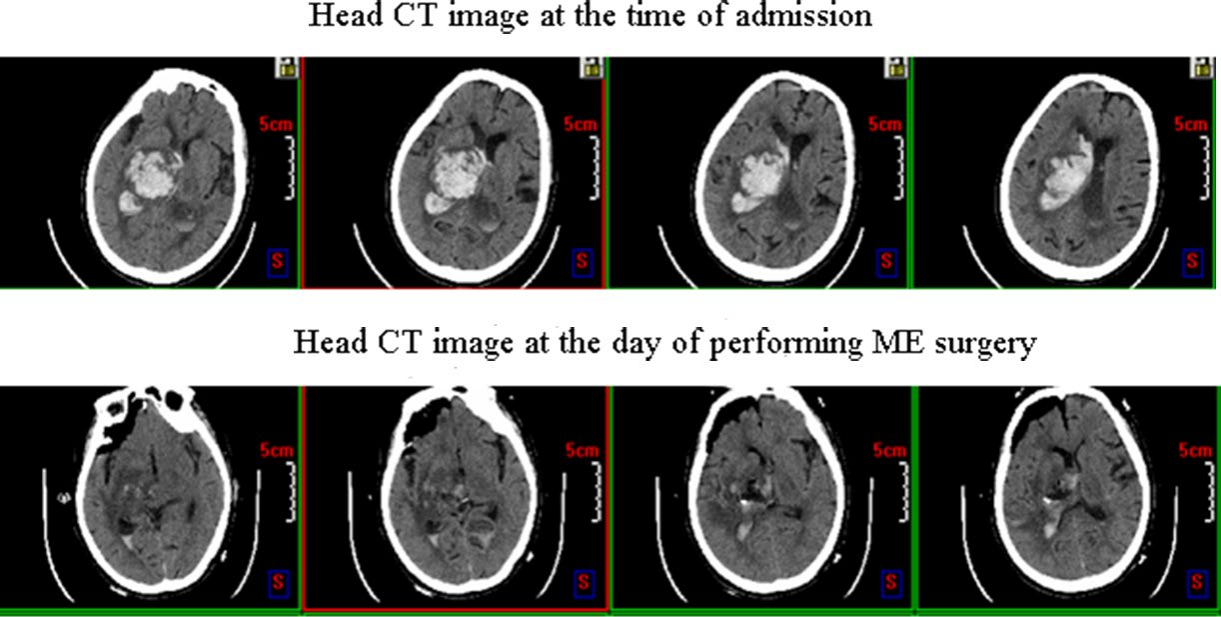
Head CT images of intracranial hematoma volume in the patient at 6 hr after administration and at the day that treated with minimally invasive stereotactic puncture therapy combined with external ventricular drainage (MISPT+EVD,ME)
